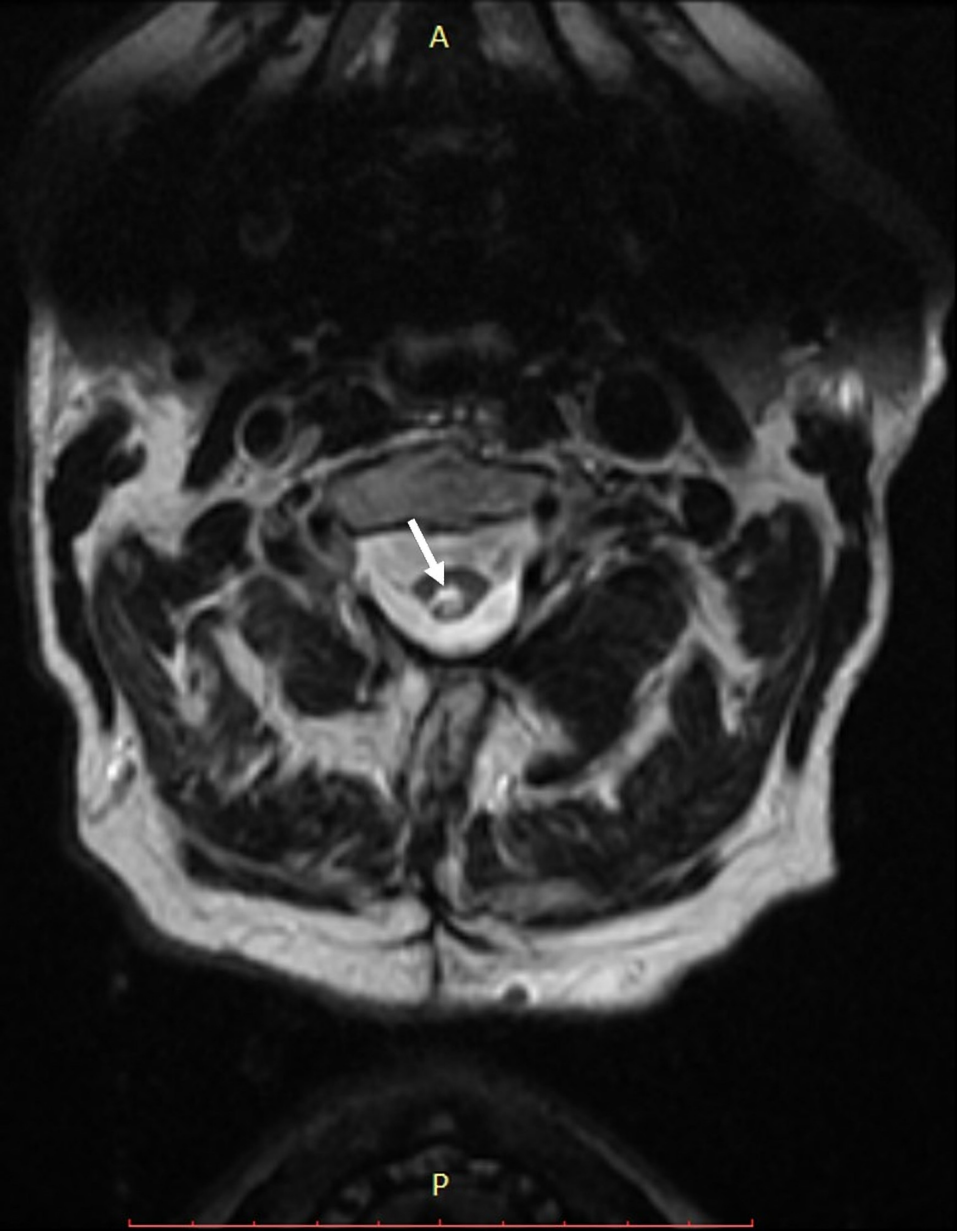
Axial T2-weighted magnetic resonance imaging (MRI) at the level of C2 Showing hyperintense fluid in the spinal cord (white arrow) consistent with a syrinx.
